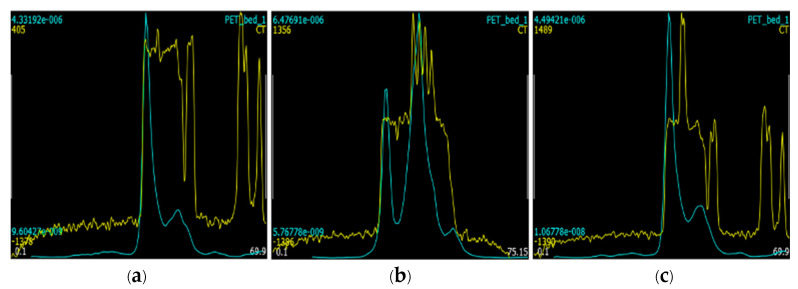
Intensity profiles of (a) trans-axial, (b) coronal, and (c) sagittal PET (blue) and CT (yellow) images at the selected slice positions as shown in Figure 6. PET and CT intensities are normalized for display. The additional numbers in the figures are the maximum and minimum intensity profile values of PET (blue) and CT (yellow) and the axis range (white).
